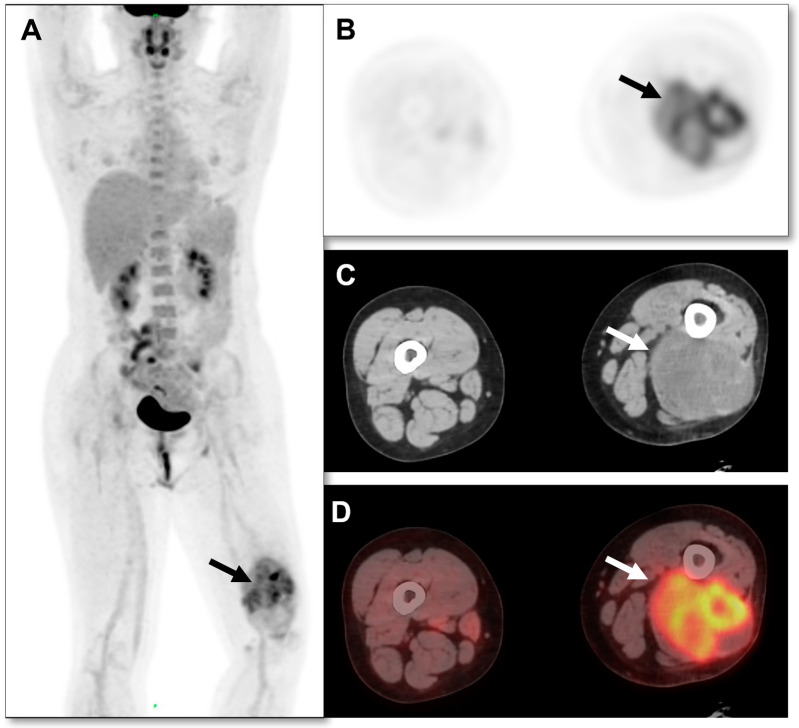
The maximum-intensity-projection (MIP) image of ^18^F-FDG PET/CT (A) showed increased radiotracer uptake in the posterior muscle layer of the left lower thigh (arrow). The MIP image does not show the presence of nodal or distant metastases. Axial images of PET (B), CT (C), and PET/CT fusion (D) showed soft tissue density mass in the posterior muscle of the left lower thigh with increased diffuse FDG radiotracer uptake and uneven distribution of lesion density and FDG (arrow; SUVmax 6.8; Size, 7.8 × 9.9 × 14.3 cm). No clear abnormal high radiotracer uptake was observed in other parts of the patient. Based on ^18^F-FDG PET/CT findings, the lesion was considered a primary malignant tumor.
